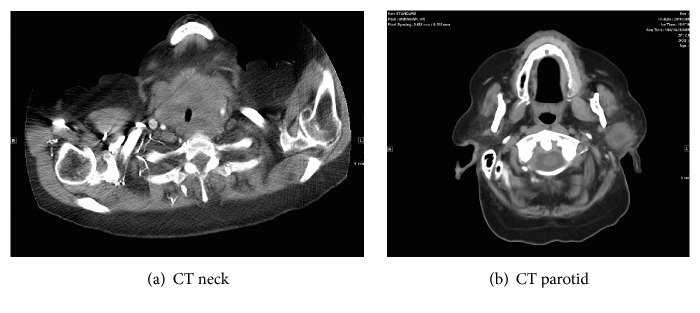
CT images of neck mass (a) demonstrating diffuse thyroid mass with tracheal luminal compression and left parotid mass (b) with central necrosis.
